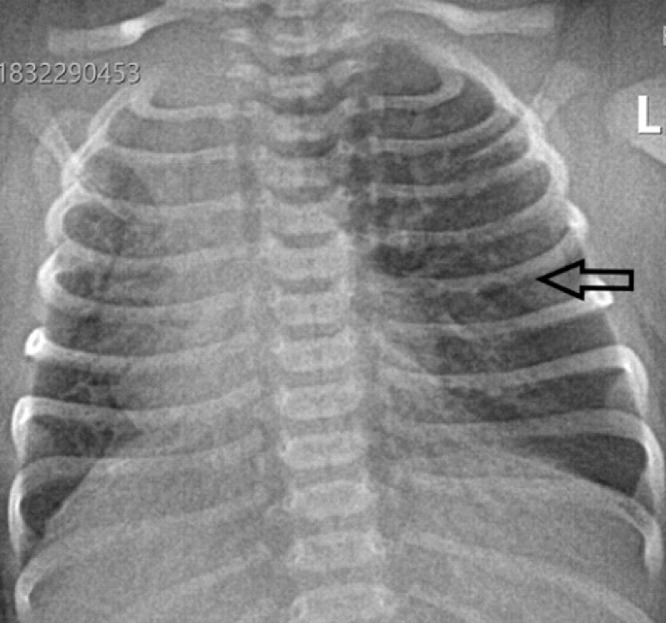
Frontal chest radiograph showing hyperinflation of the left lung (arrow) with mediastinal shift to the right side.
